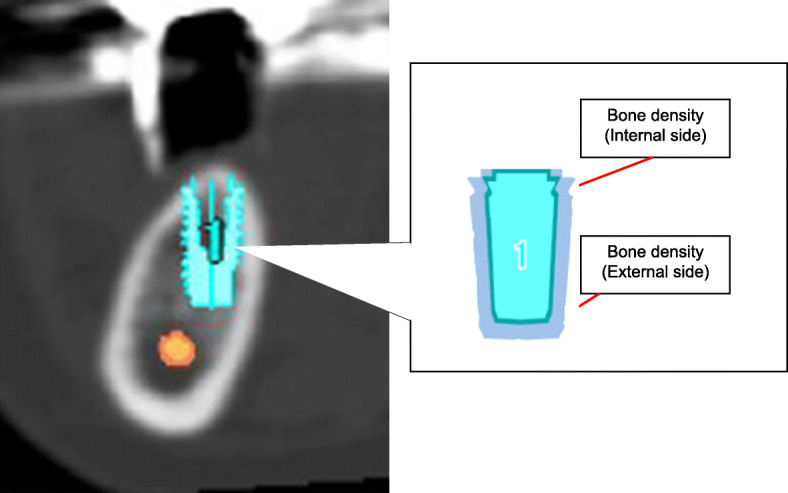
Measurements on computed tomography (CT) images. The imaging data are stored in Digital Imaging and Communications in Medicine format and loaded into an implant planning software program (Simplant®, Dentsply Implants, Leuven, Belgium). Buccopalatal or buccolingual cross-sectional CT slices are used to measure the bone density at the implant placement sites in Hounsfield units. The mean internal and external side values are used as the bone densities
